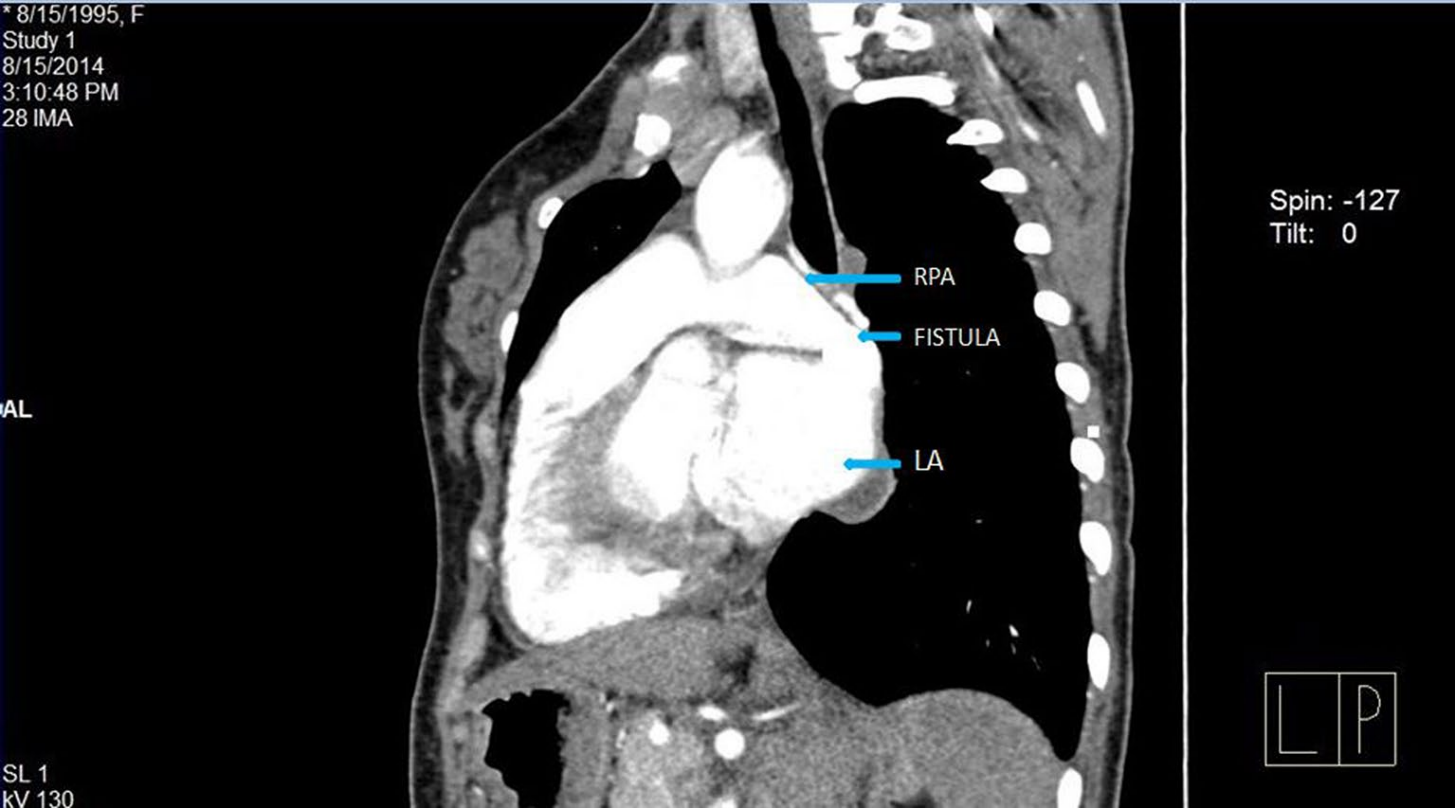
Contrast enhanced CT chest showing shunt between right pulmonary to left atrium (oblique view)
